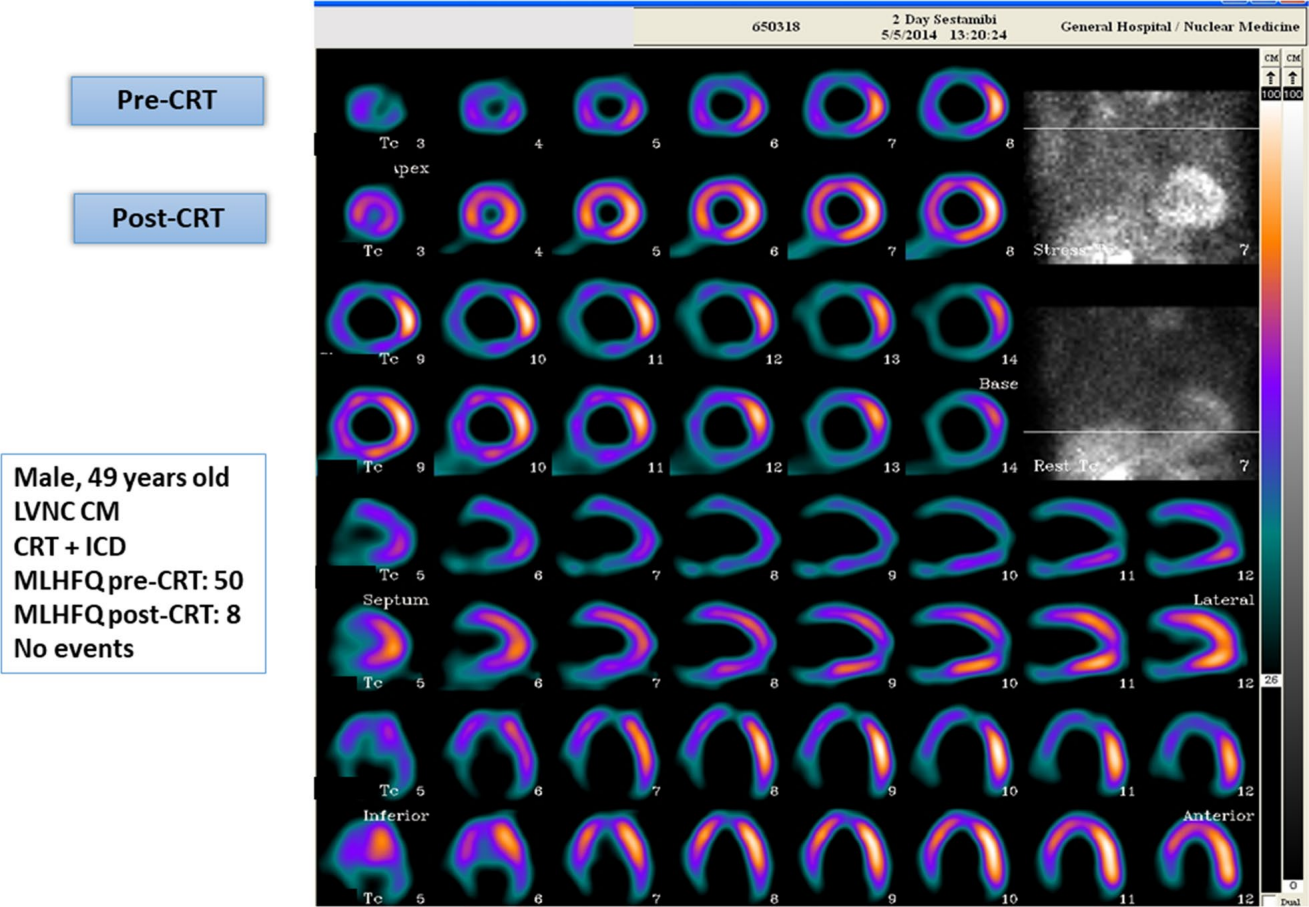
^99^m Technetium-MIBI gated-SPECT MPI in a 49-y.o. male patient with diagnosis of left ventricular non-compaction cardiomyopathy. Perfusion images comparing pre- and post-CRT. *CRT* cardiac resynchronization therapy, *ICD* implantable cardioverter defibrillator, *LVNC CM* left ventricular non-compaction cardiomyopathy, *MLFHQ* Minnesota Living with Heart Failure Questionnaire
